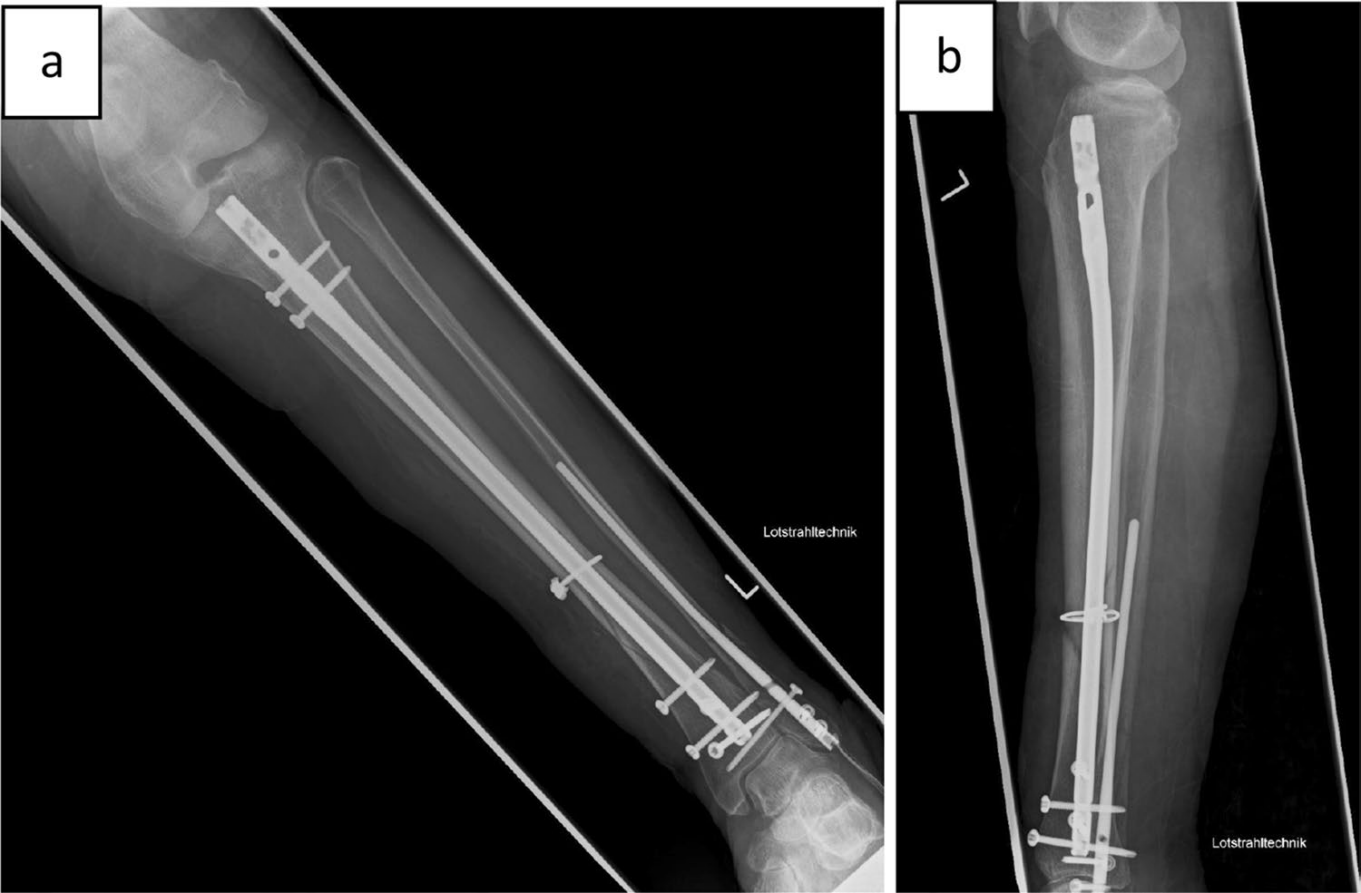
Postoperative X-ray after minimally invasive cerclage and nail osteosynthesis of the tibia (Expertnail, DePuy Synthes Companies, Oberdorf, Switzerland) and fibula (Vitus Fi, Marquardt Medizintechnik GmbH): ap (a) and lateral (b) view. Mobilization is allowed under full weight bearing
